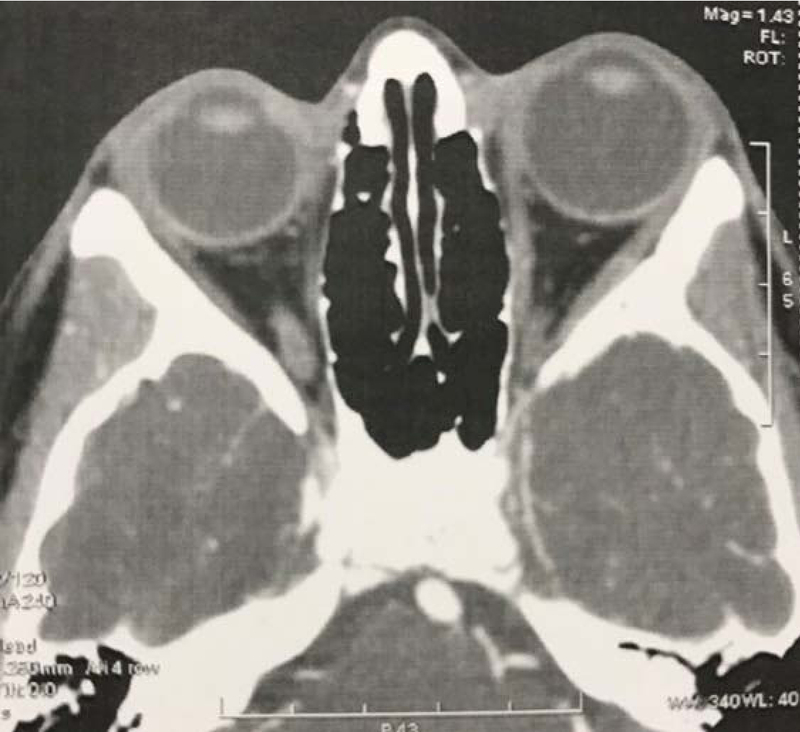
Axial computerized tomography (CT) scan shows left proptosis and different globe size.
